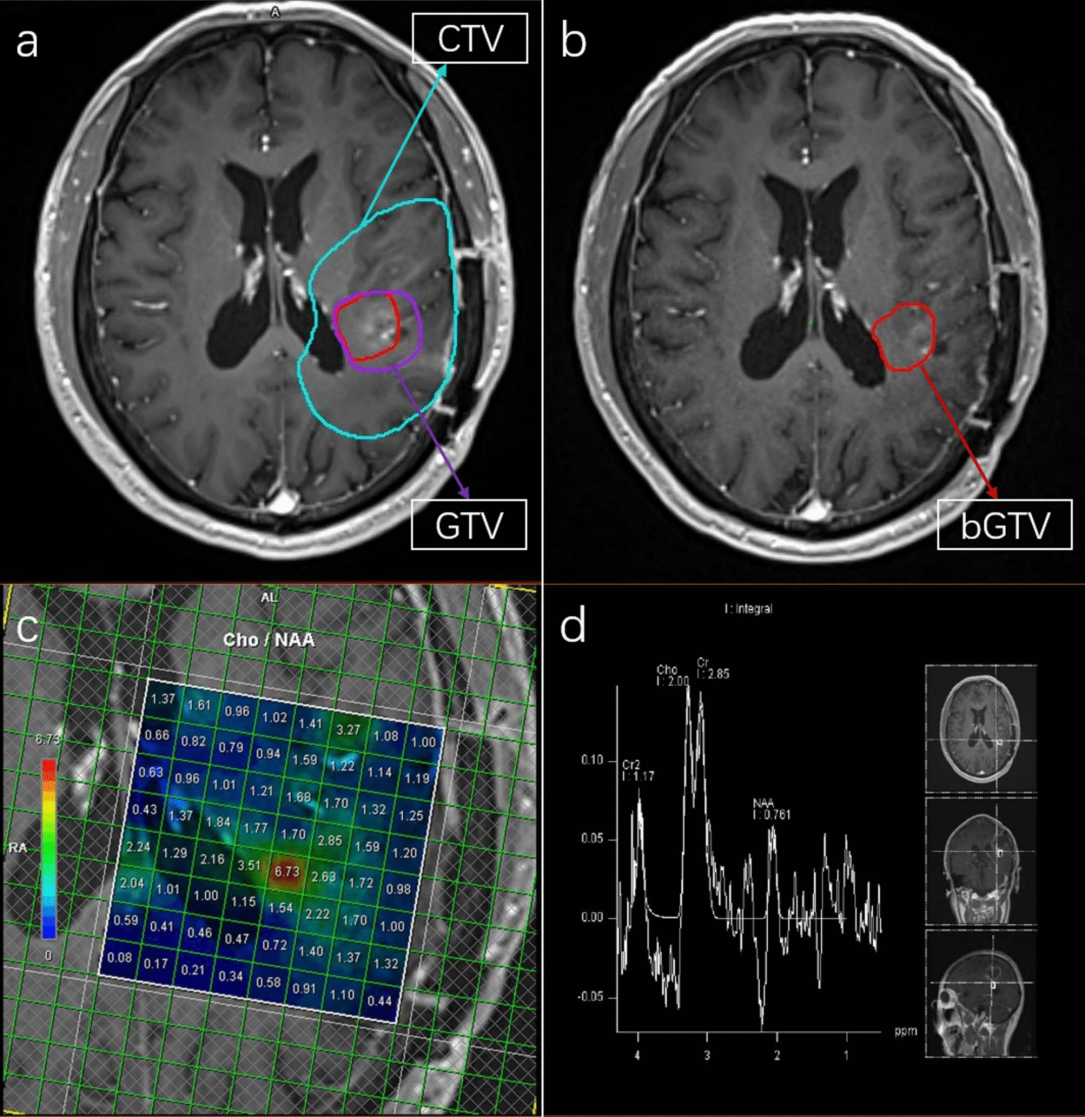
High-risk tumor regions as determined by MRS after standard radiotherapy in patients with GBM: (a) T1-weighted sequence before radiotherapy; (b) bGTV in T1-weighted sequence after a 60 Gy dose; (c, d) regions of interest showing the Cho/NAA ratio.
